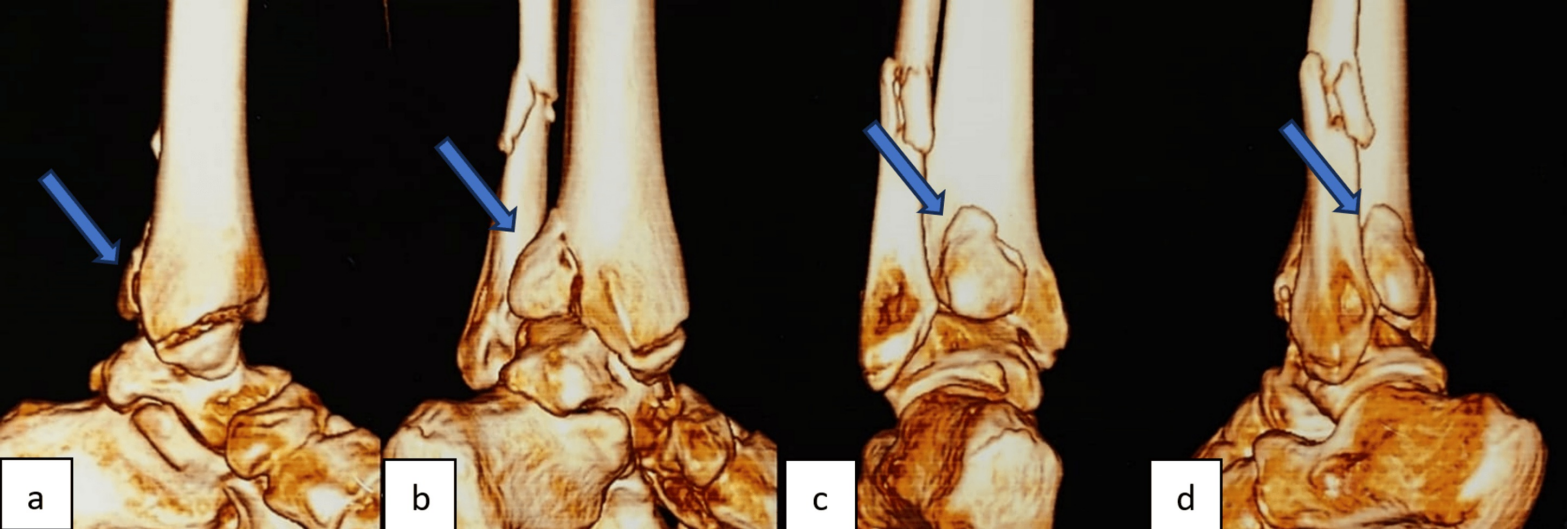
3D CT of an ankle showing posterior malleolus fracture fragment (a), (b), (c), and (d) showing different viewing angles of posterior malleolus fracture in 3D CT. CT: Computed tomography
